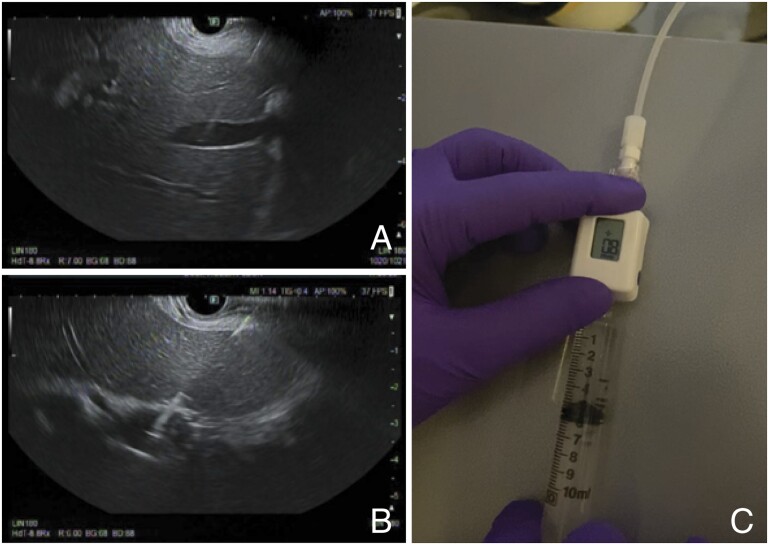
Endoscopic ultrasound (EUS)-guided portal pressure gradient measurement. (A) A 25-gauge needle is used for transhepatic EUS-guided access to the left hepatic vein. (B) The 25-gauge needle is used for transhepatic EUS-guided access to the portal vein. (C) The compact manometer is placed at the midaxillary line to establish the phlebostatic axis.
